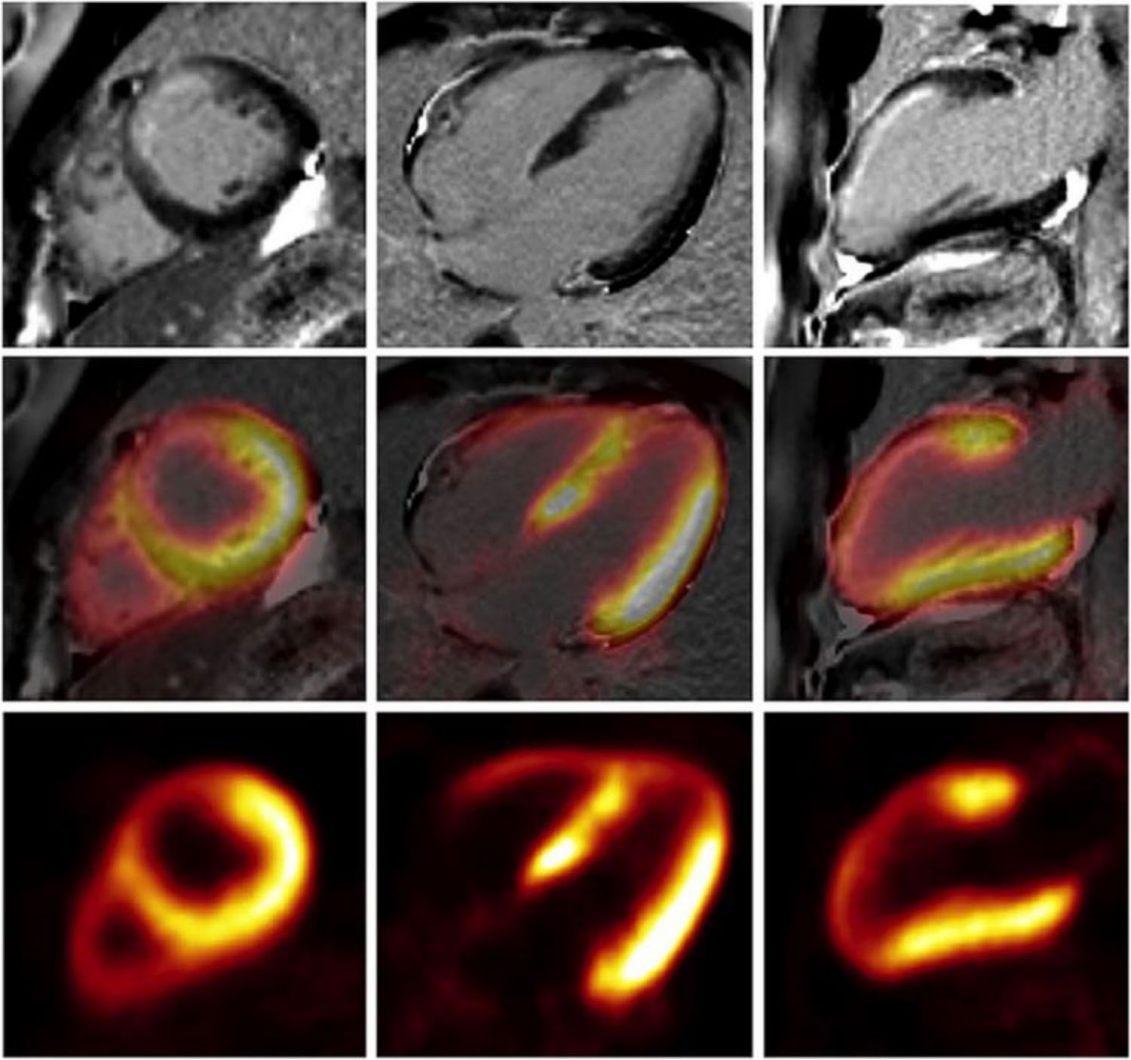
Viability imaging using FDG PET and LGE MRI in a patient with a scar of the anterior wall. Reduced glucose metabolism and late gadolinium enhancement is present in the area of scarring in the anterior wall (reprinted with permission from [161])
